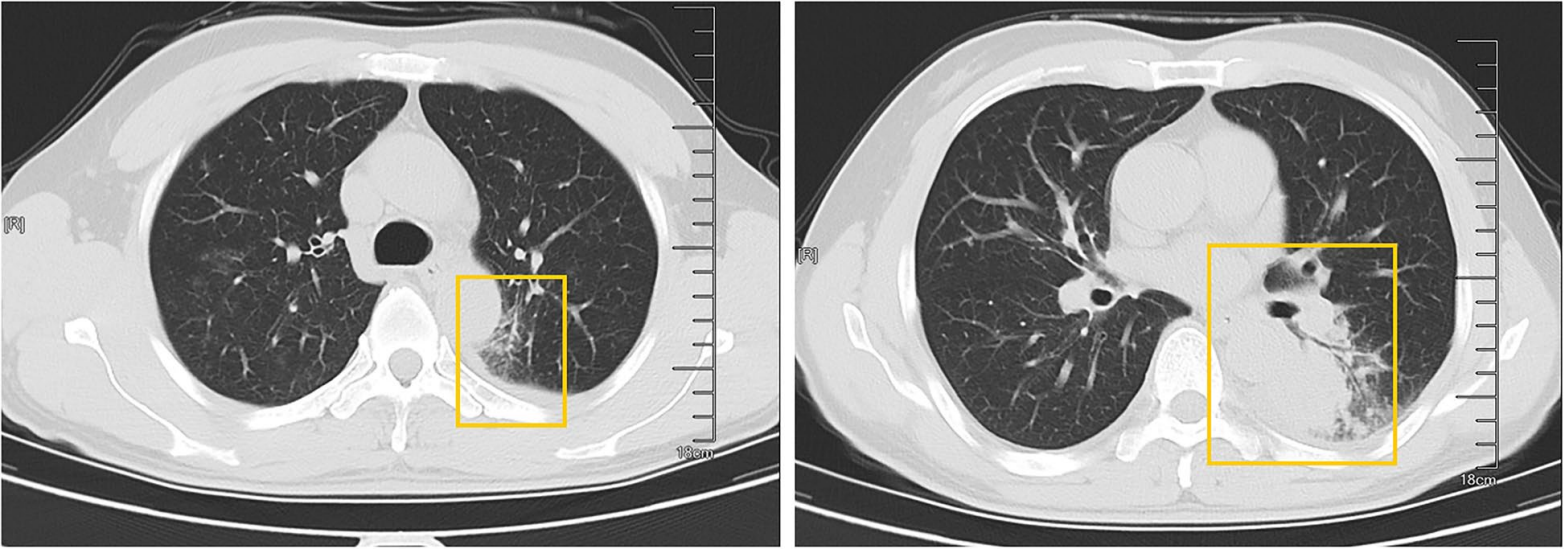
The patient’s pulmonary CT scan shows patchy and nodular lesions accompanied by a small amount of pleural effusion, later confirmed to be MDR-PTB
